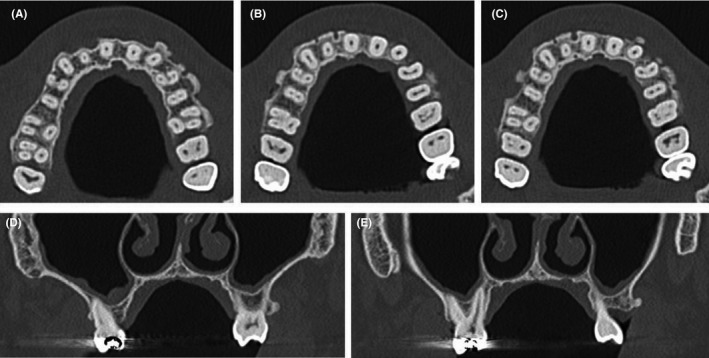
(A‐E) The cone beam computed tomography showed multiple proliferating osseous lesions with irregular appearance and dimension emerging from the buccal cortical plate of the maxilla without signs of teeth involvement
